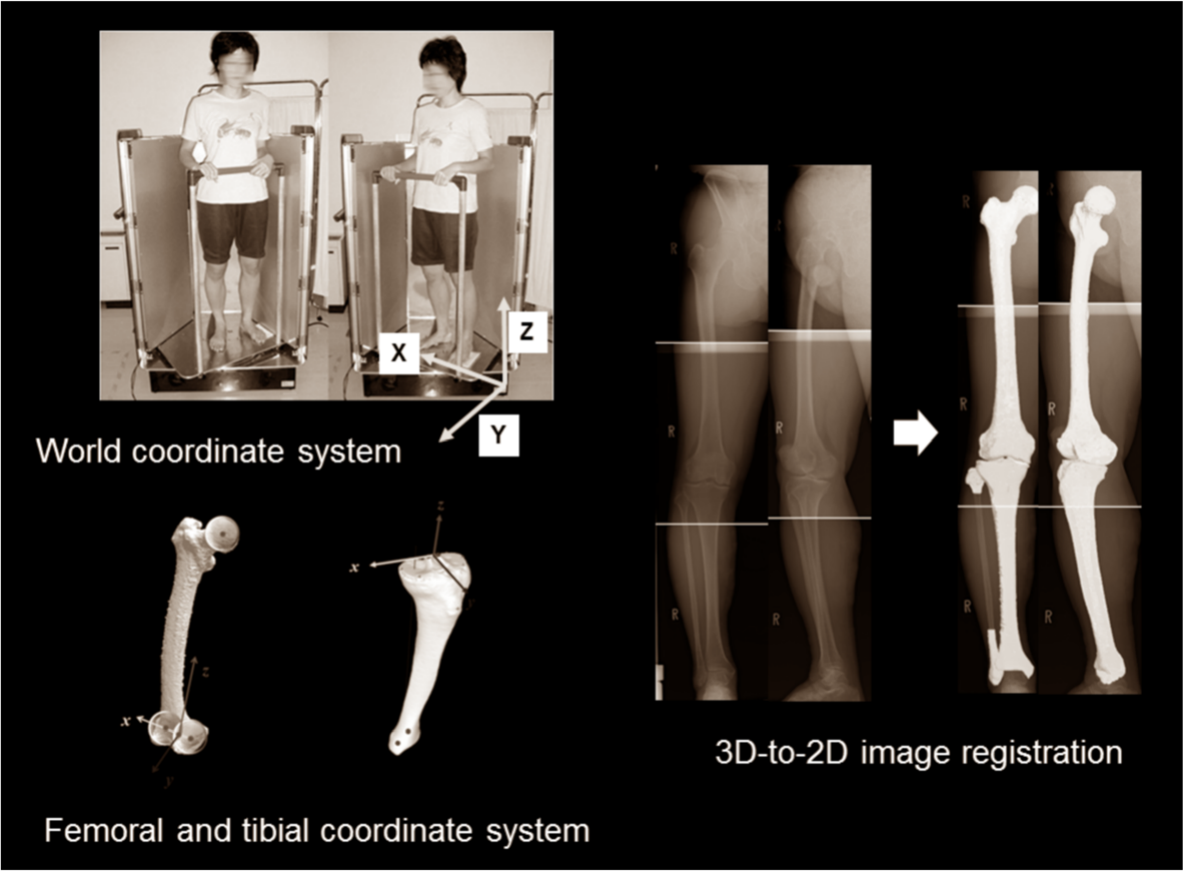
The 3D lower extremity alignment assessment system under WB conditions based on biplanar long lower extremity radiographs, applying the 3D-to-2D image registration techniques. 3D bone models and the anatomic coordinate system of the femur and tibia were reconstructed. The 3D bone model included the definitions of the parameters in the bony morphology and whole lower extremity alignment. To assess the 3D parameters “in the standing position” under WB conditions, biplanar long lower extremity radiographs were obtained in the standing position. Applying the 3D-to-2D image registration technique, projected outline points of each 3D model were the finite edge points of the 2D shadow created from the projections of all visible triangular surfaces of the 3D model. To precisely assess the position and angle to the ground, the world coordinate system was reconstructed
